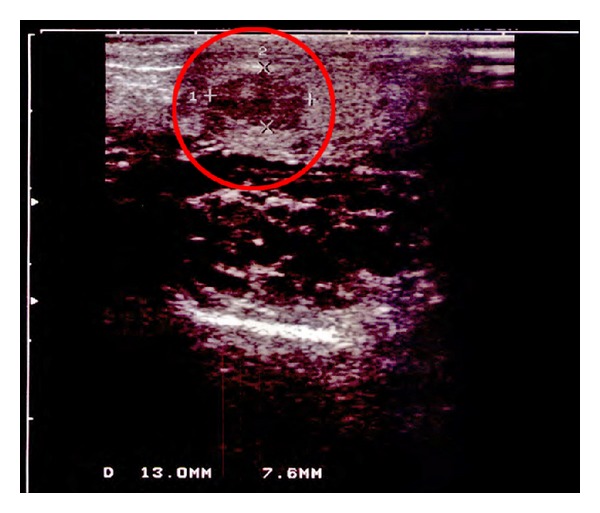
Tumor (red circle) and high-grade varicocele in sonography.
